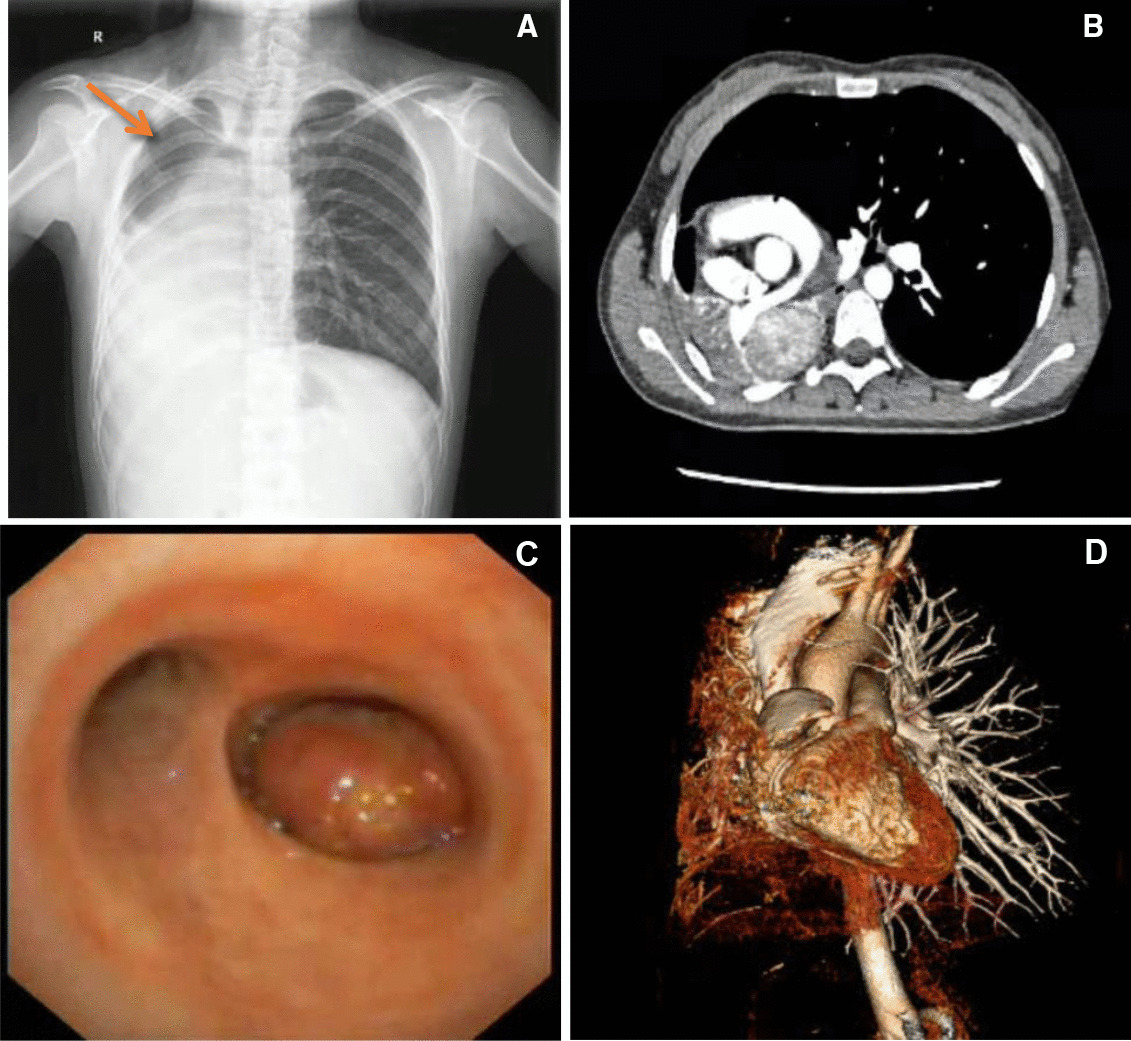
Preoperative imaging of the large acinic cell carcinoma of right lung. A Preoperative chest radiograph showed that the mediastinum was completely deviated to the right thorax and the right lung was completely atelectasis. Arrow: part of the left lung tissue that deviate to the right thorax. B CT scan showed the right lung was completely atelectated, with tumor tissue invading the right hilum structure. C Fiberoptic bronchoscopy revealed a tumor with obstruction of the right main bronchus and protrusion of adjacent organs. D 3D-reconstruction showed The vascular anatomical structure of the right hilum is completely disorganized and difficult to recognize
